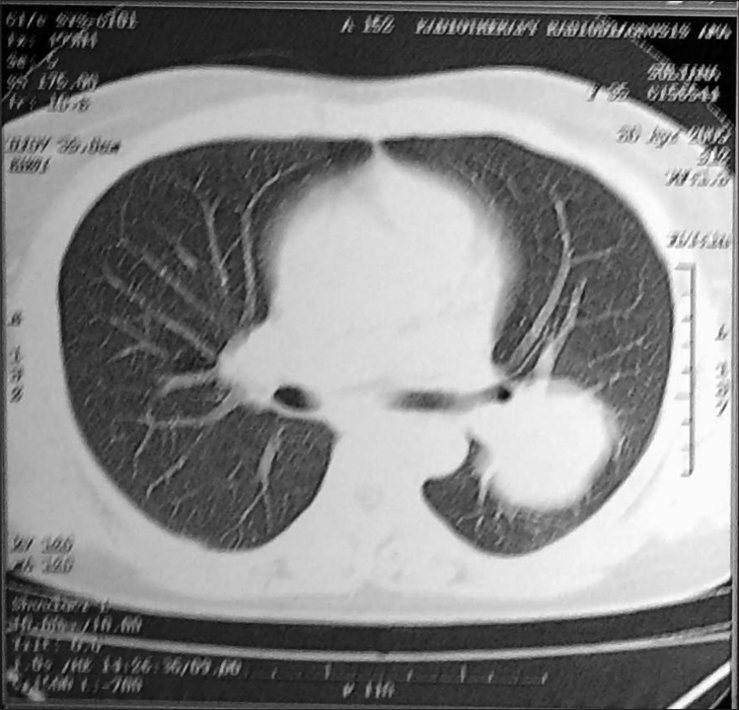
Computed tomography (CT) scan shows a well defined mass
